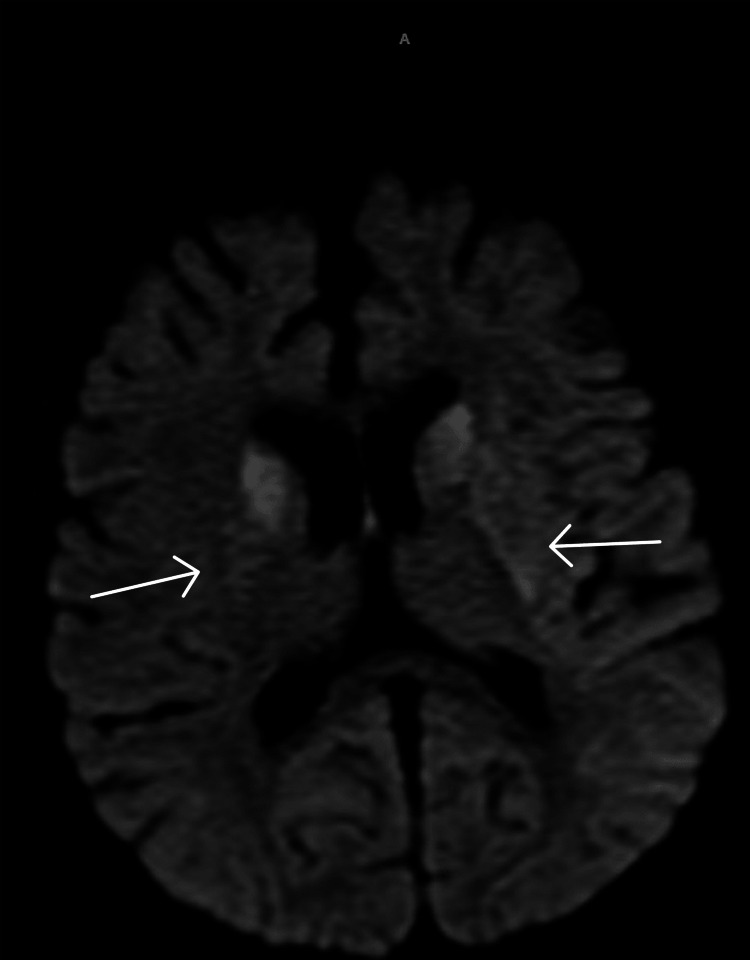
Diffusion-weighted image MRI showing bilateral symmetric areas of diffusion restriction mainly involving the basal ganglia including the caudate nucleus putamen and globus pallidus pallidum.
